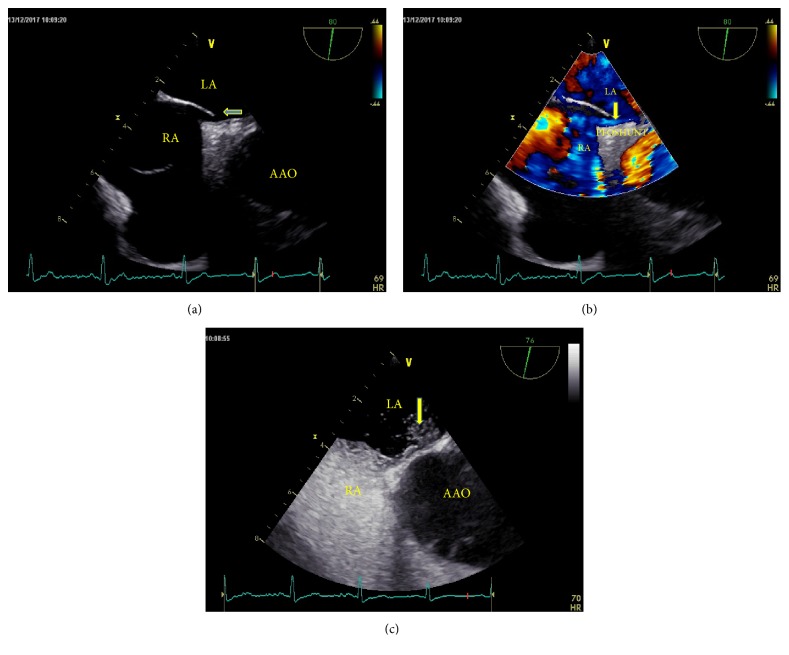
(a) Two-dimensional TEE displaying a “slit-like” channel between the left and right atria, revealing the presence of a PFO. (b) Doppler color flow imaging showing spontaneous PFO-left-to-right shunt. (c) TEE bubble study showing the microbubbles passing through the PFO.
